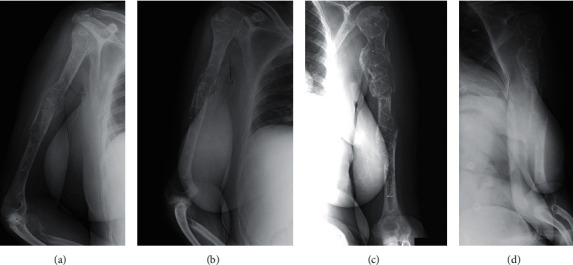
(a, b) Two radiographs showing bone healing of the pathologic fracture of the right humerus at two months of follow-up. (c, d) Two radiographs showing bone healing of the pathologic fracture of the left humerus at two months of follow-up.
